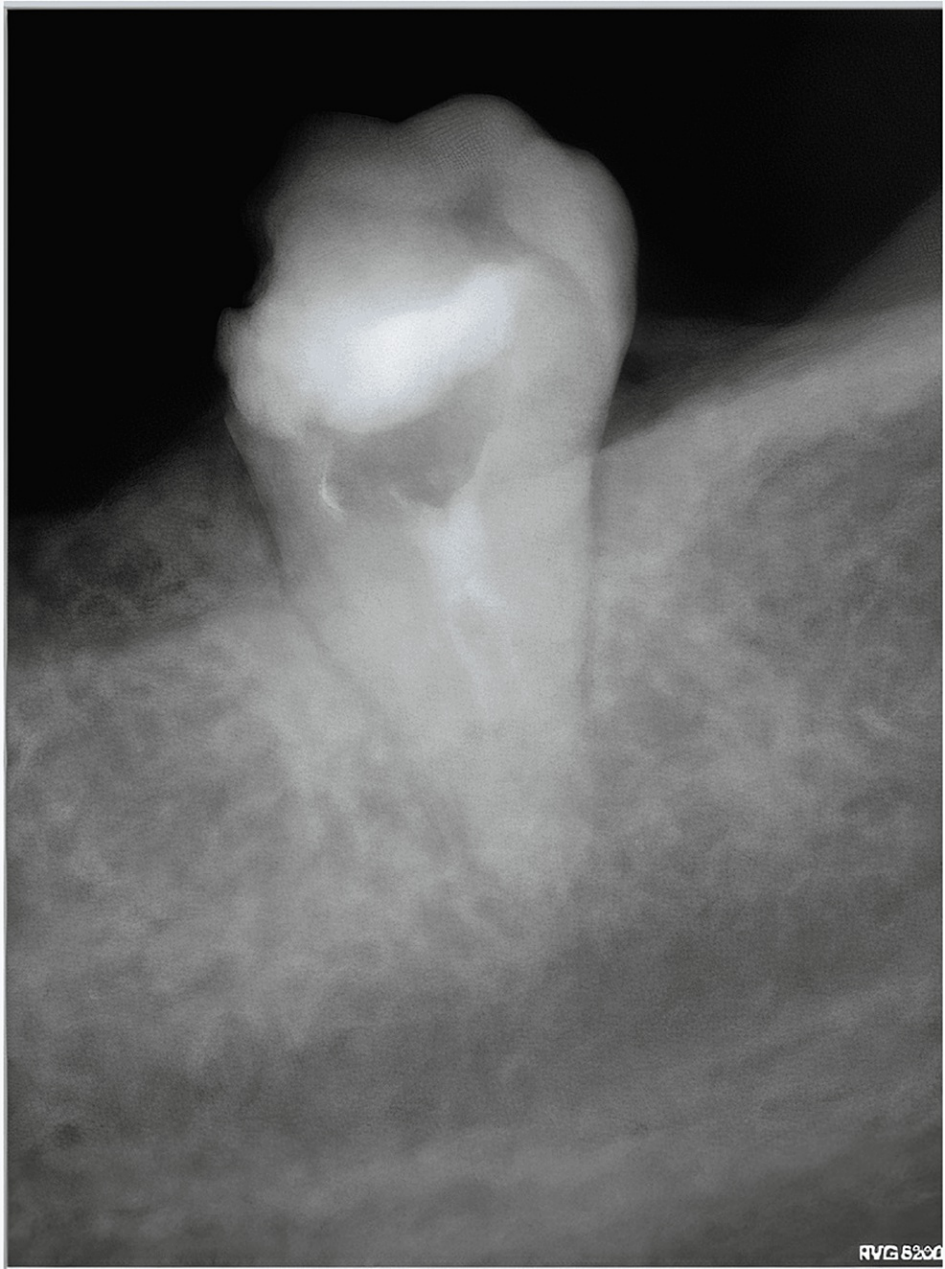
Pre-operative intraoral periapical radiograph demonstrating the radiographical features of tooth number #37 The presence of intracanal medicament is noticed in the radiograph
